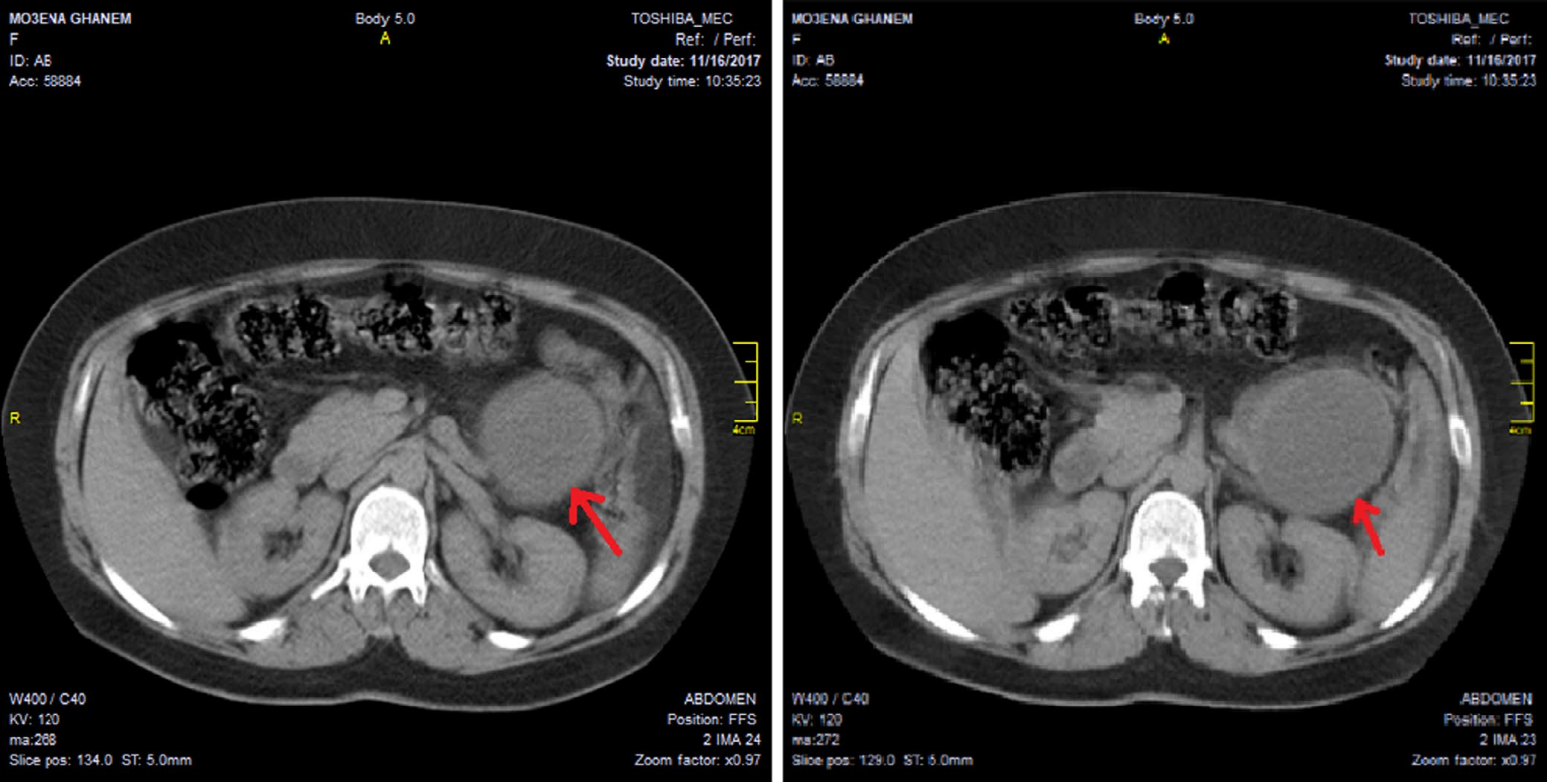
Noncontrast CT scan revealed unilocular cystic lesion in the tail of the pancreas attached to the spleen
